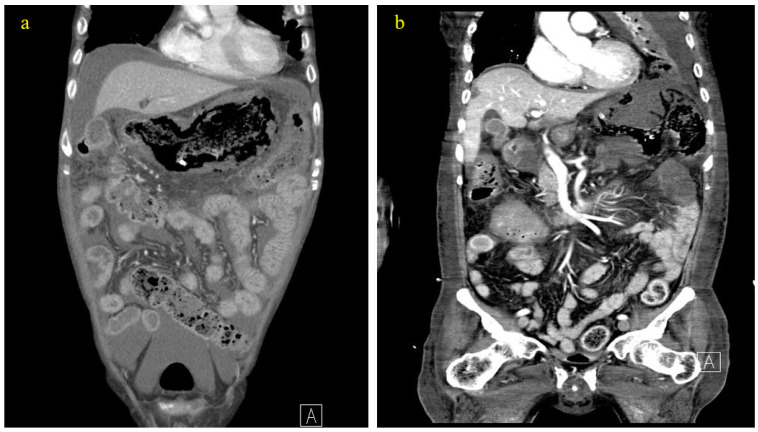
Abdominal computerized tomography (CT) show air around the stomach with ascites on the 20th hospital day (a), indicating gastric perforation. The post-operational CT shows an increased extent of ischemia/infarction in multiorgan in the abdomen, including small bowel, colon, liver, pancreas, and spleen (b).
